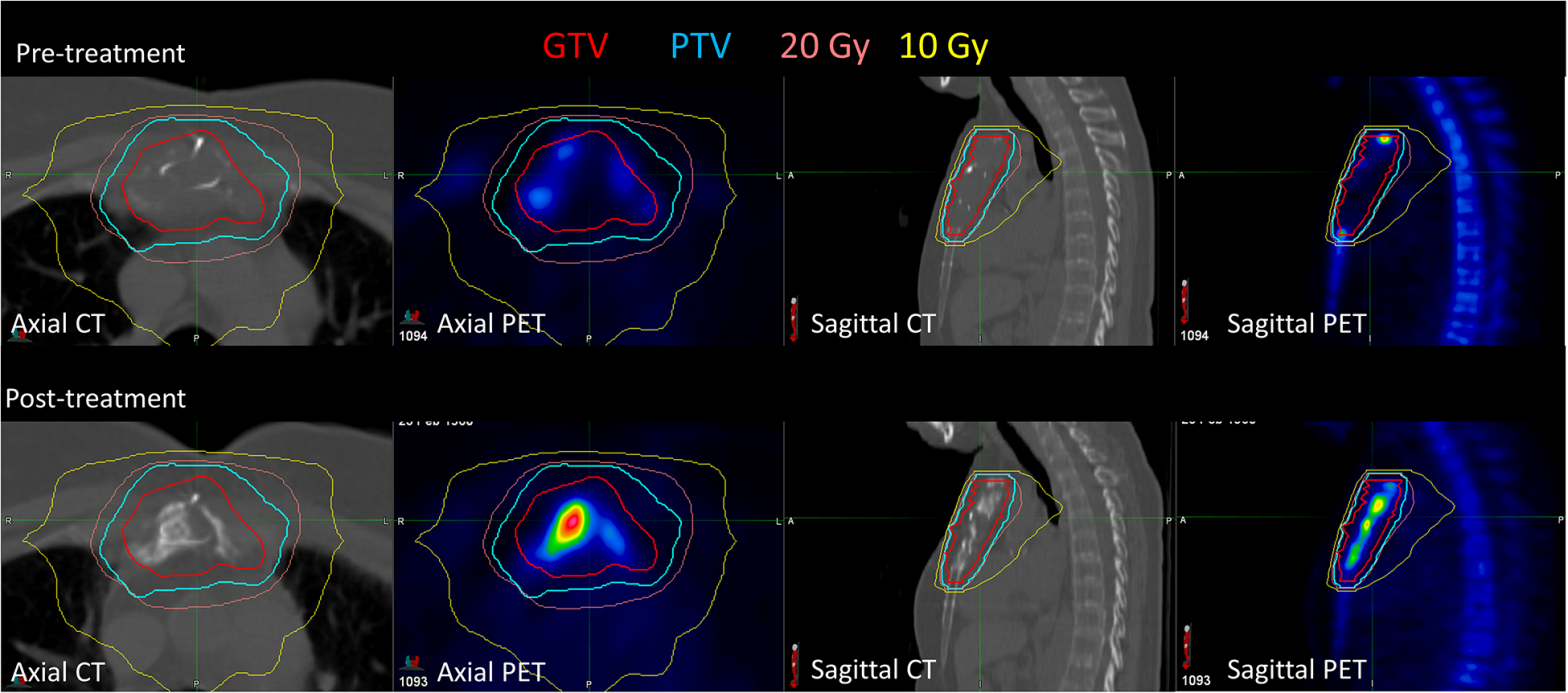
Pre-treatment and post-treatment CT and [^18^F]NaF PET for patient 7, who had a sternal metastasis treated. After treatment, [^18^F]NaF SUV_max_ decreased from 36.4 to 30.8, and SUV_mean_ increased from 3.5 to 3.9, corresponding to bone regeneration.
